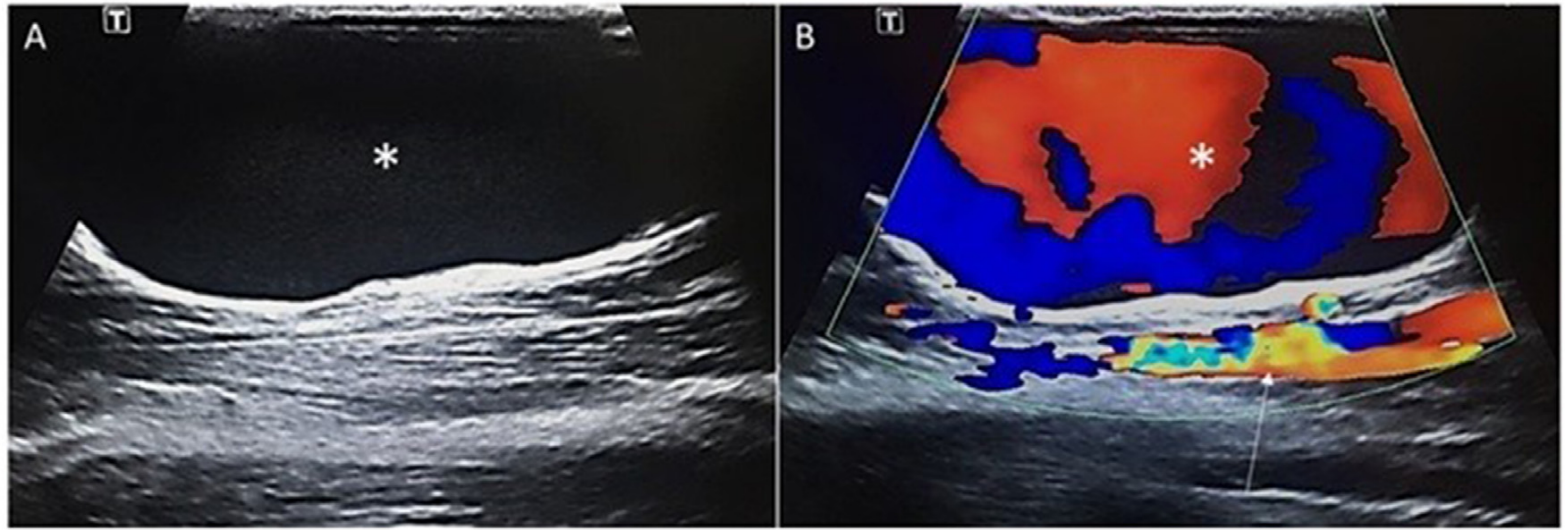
Grayscale (A) and color Doppler (B) ultrasound of the basilic vein demonstrates fusiform aneurysmal dilatation of basilic vein (asterisk) is noted with a slow turbulent flow in color Doppler study. The ulnar artery is seen deep to the Aneurysmally dilated basilic vein (arrow).
